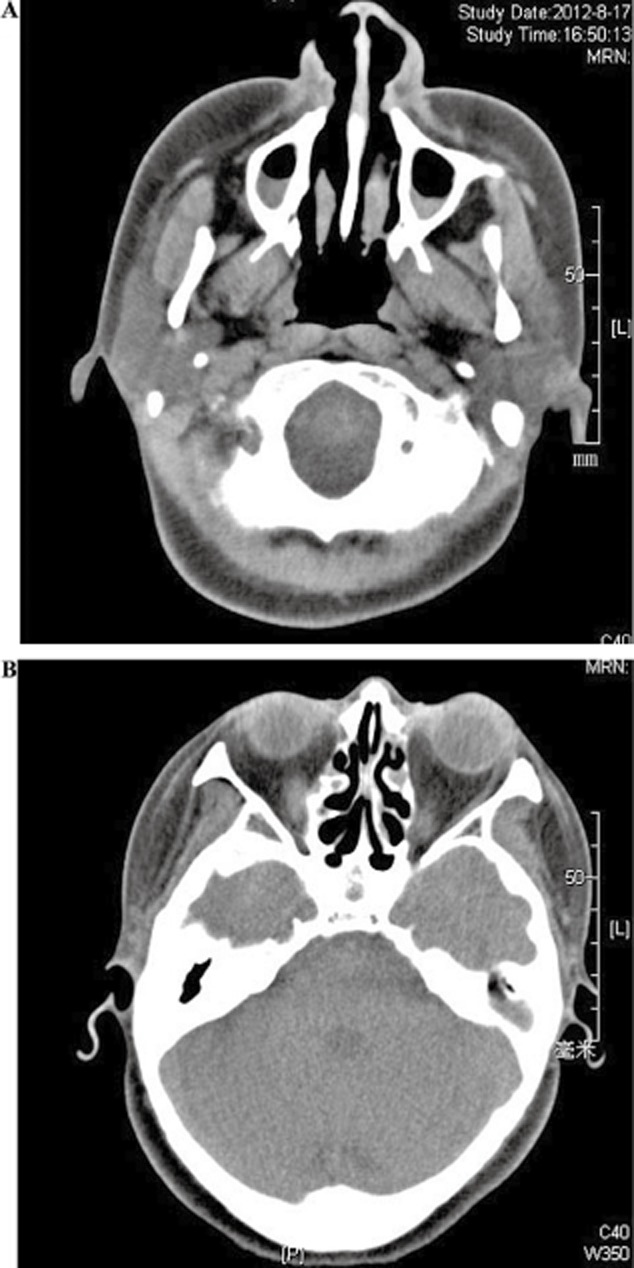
Computed tomography (CT) images of the sinuses showed chronic maxillary sinusitis and (B) ethmoid sinusitis.
